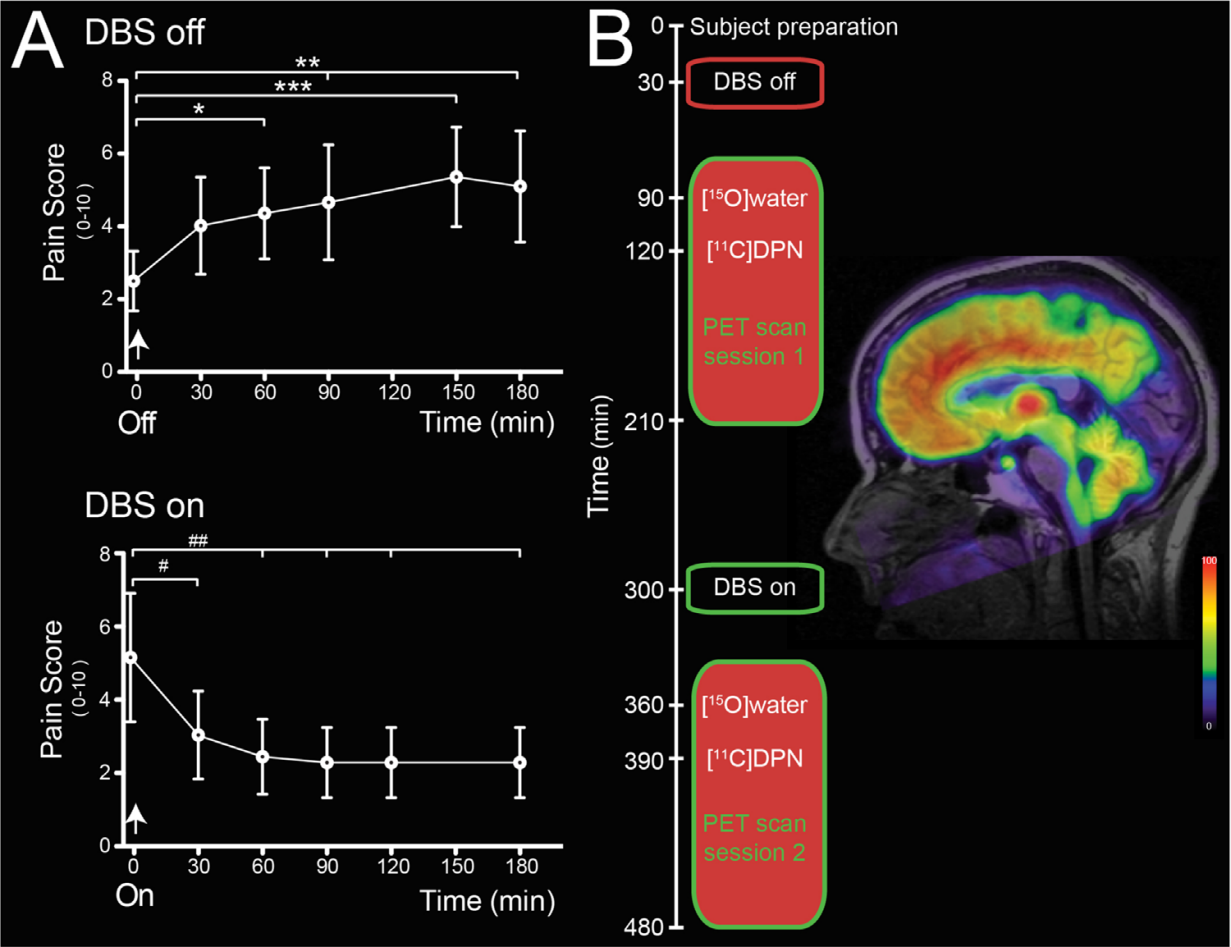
Acute analgesic effects of DBS and change in [^11^C]DPN binding. (A) Change in pain scores with DBS stimulation assessed during home trial. The analgesic effect of stimulation was almost completely lost 60 min after inactivation of DBS (pain VAS increased from 2.5±0.8 to 4.4±1.3, *p*<0.05, *n*=5) to reach a plateau level of pain that continued for more than two hours. On re-commencing PAG stimulation the analgesic effect was evident within 30 min (pain VAS reduced by 41% from 5.1±1.8 to 3.0±1.2) and this analgesic action was maintained for the rest of the test period. (rm-ANOVA with Dunnett's posthoc tests, *,# – *p*<0.05; , ## – *p*<0.01, *,### – *p*<0.001.). (B) Timeline of the sequential [^15^O]water and [^11^C]DPN scans with the first scanning session following 60 min after DBS was stopped and the second commencing 60 min after DBS was reinstated. Shown alongside is a representative sagittal parametric image of the volume of distribution (V_T_) for [^11^C]DPN overlaid on T_1_-weighted MRI image for a single subject (h00635) with DBS off. The image shows a characteristic distribution of the opioid ligand with high binding in the thalamus, midbrain and in several cortical regions (such as prefrontal and cingulate) but low binding in the occipital cortex and the pontine nucleus.
